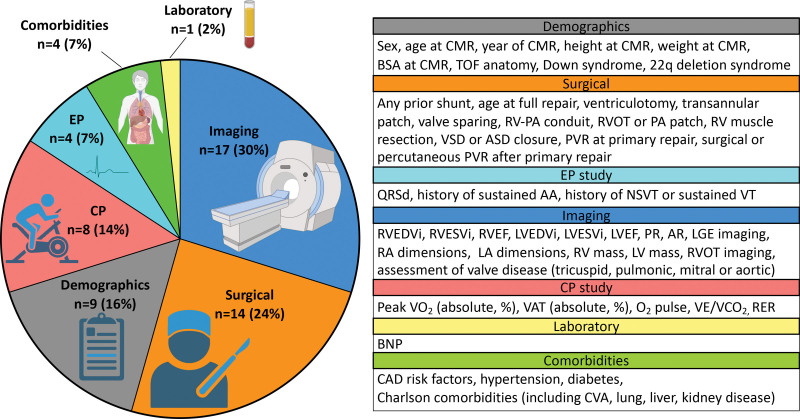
Clinical and imaging variables incorporated into the machine learning model (n=57 variables). AA indicates atrial arrhythmia; AR, aortic regurgitation; ASD, atrial septal defect; BNP, brain natriuretic peptide; BSA, body surface area; CAD, coronary artery disease; CMR, cardiovascular magnetic resonance imaging; CP, cardiopulmonary exercise study; CVA, cerebrovascular accident; EDVi, end-diastolic volume indexed; EF, ejection fraction; EP, electrophysiology study; ESVi, end-systolic volume indexed; LA, left atrium; LGE, late gadolinium enhancement; LV, left ventricular; NSVT, nonsustained ventricular tachycardia; PA, pulmonary artery; PR, pulmonary regurgitation; PVR, pulmonary valve replacement; QRSd, QRS duration; RA, right atrium; RER, respiratory exchange ratio; RV, right ventricular; RVOT, right ventricular outflow tract; TOF, tetralogy of Fallot; VAT, ventilatory anaerobic threshold; VE/VCO_2_, minute ventilation/carbon dioxide production relationship; VO_2_, aerobic capacity; VSD, ventricular septal defect; and VT, ventricular tachycardia (portions of this figure were created with Biorender.com)
